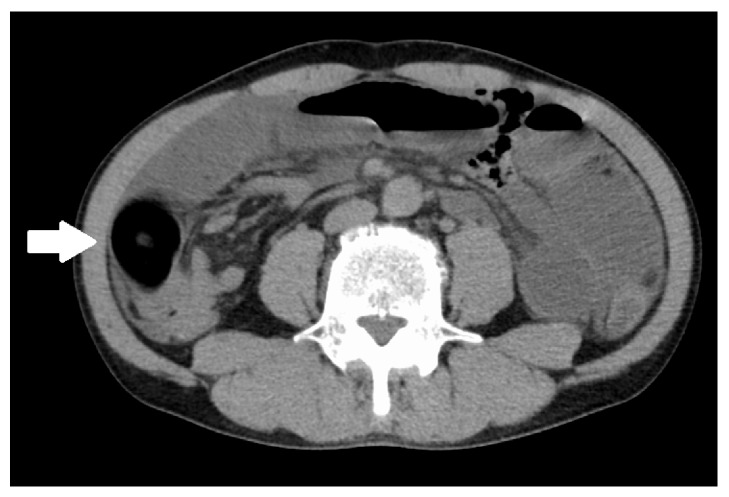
Computed tomography revealed intestinal obstruction by a 3 × 4 cm foreign body (white arrow) within the right lower abdominal cavity.
